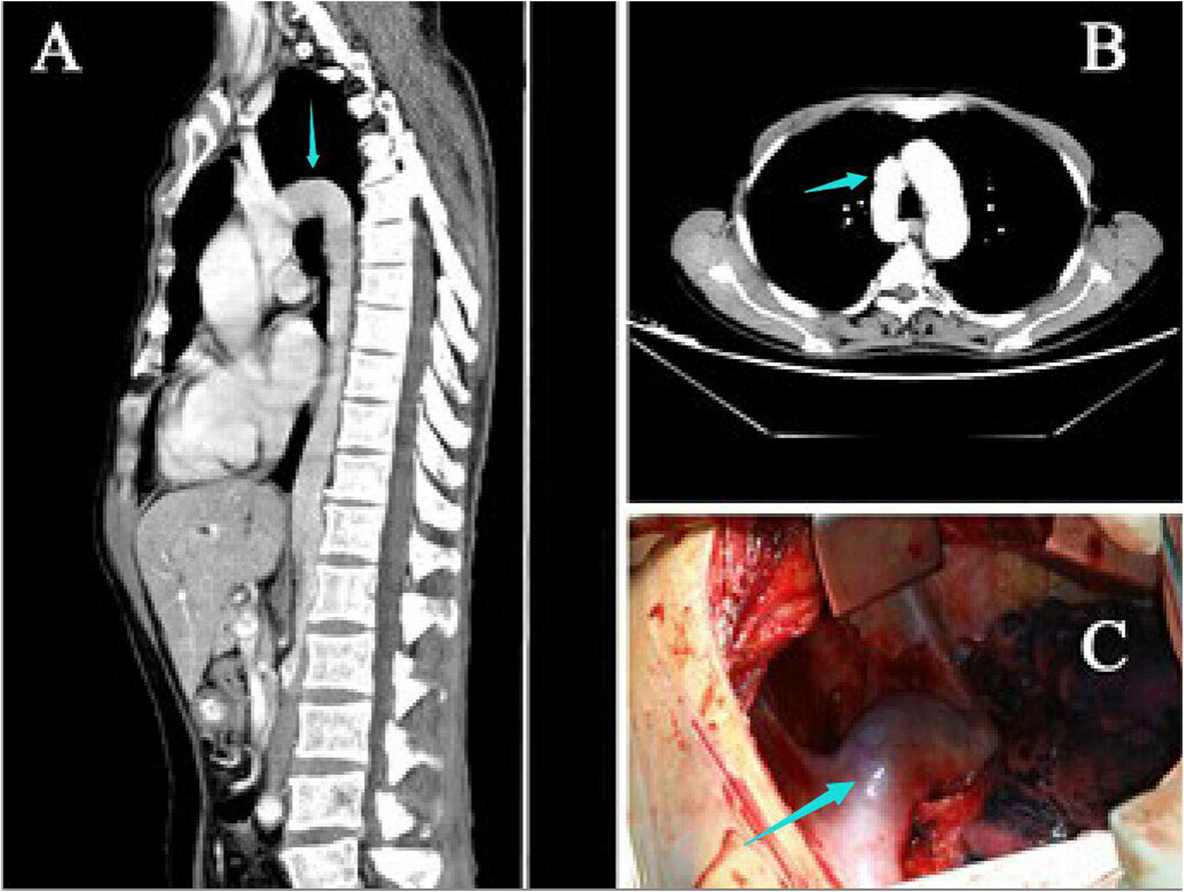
The enlarged azygos vein continuation of the inferior vena cava. a The azygos vein continuation of the IVC. b The CT scan revealed an enlarged arch of the azygos vein across the aortic arch. c Macroscopically, the diameter of the azygos vein was approximately 2.5 cm
